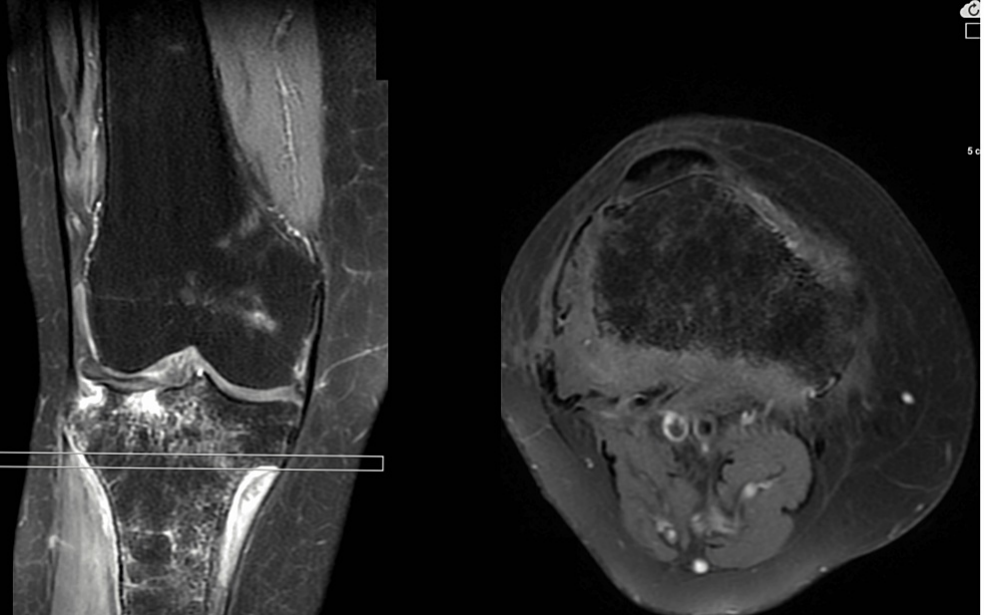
On the coronal and axial T2-weighted MRI images of the patient, hypointense osteolysis in the proximal fibula and medial and lateral resorptive changes with bone marrow edema in the tibia are observed.
